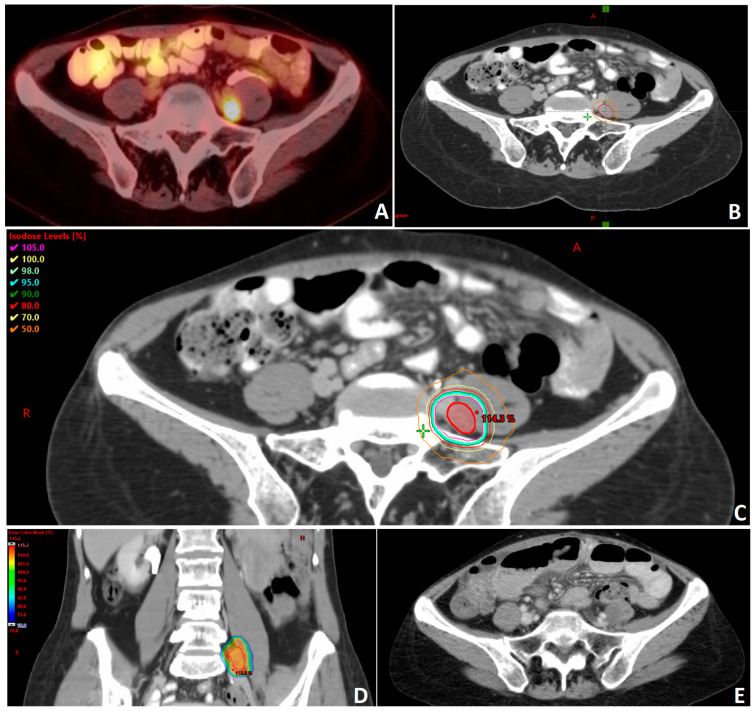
Clinical example of a patient with recurrent and oligometastatic high grade serous carcinoma of the ovary following surgery and multiple lines of systemic therapy. (A) Patient was found to have a hypermetabolic nodule medial to the left psoas muscle on positron emission tomography (PET) compatible with metastatic disease. (B) Gross tumor volume (GTV) delineation of oligometastatic nodule (red) with 0.5 mm planning tumor volume (PTV) radial expansion (orange). The treatment plan for this patient was generated to deliver a dose of 3000 cGy in 5 fractions prescribed to the PTV. The technique used in this plan is stereotactic body radiotherapy (SBRT) utilizing volumetric modulated arc therapy (VMAT) with a 10 MV beam energy. The plan is prescribed to the PTV. Axial (C) and coronal (D) images showing dose distribution. There is a max dose of 115.2% of the prescription dose with the PTV. (E) Representative axial image of follow-up computed tomography (CT) showing resolution of the treated nodule.
